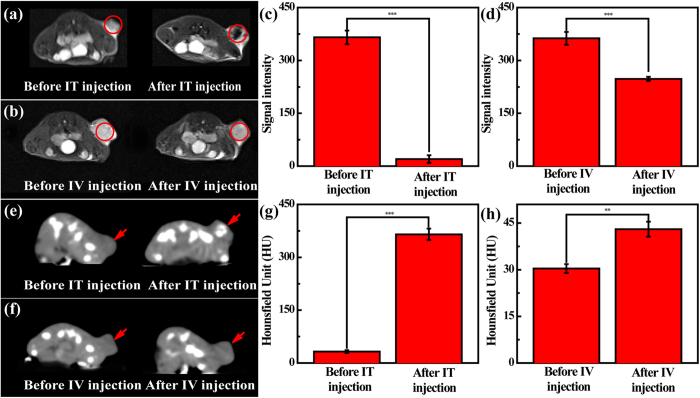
In vivo* MR/CT imaging of a xenografted tumor model. (a,b) T_2_-weighted MR images, (c,d) MR signal intensity, (e,f) CT images, and (g,h) CT value of tumors before and at 0.5 h post IT (a,c,e,g) and at 6 h post IV (b,d,f,h) injection of the Fe_3_O_4_ @ Au-PEI.Ac-PEG-FA NSs ([Fe] = 1.31 mM, [Au] = 70 mM, 0.1 mL in PBS).
